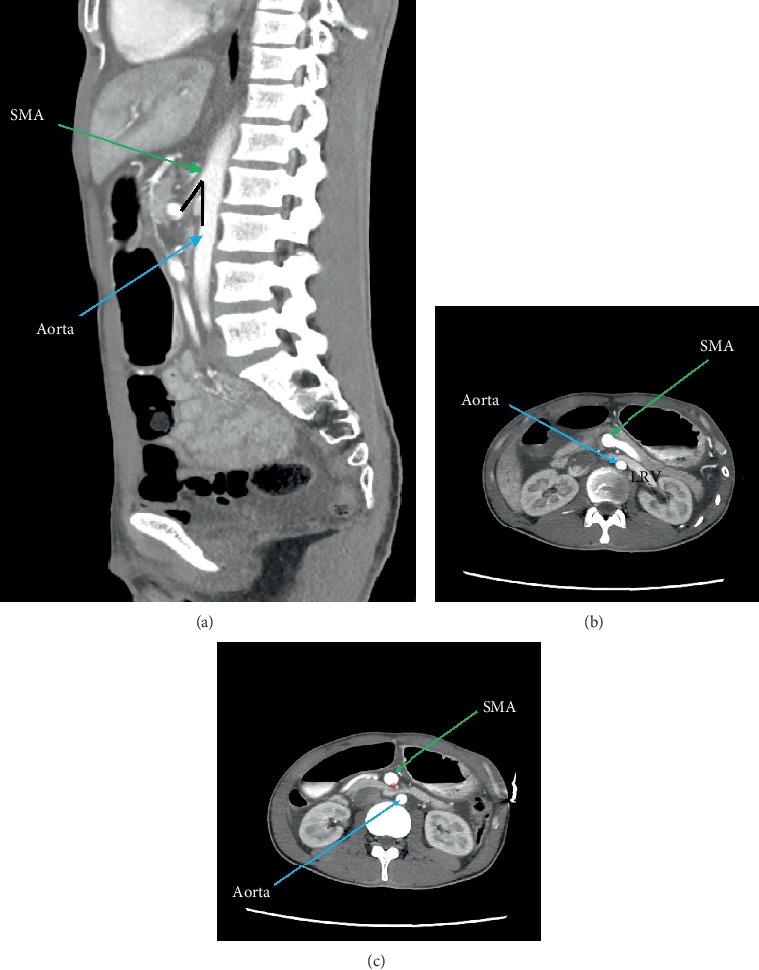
Contrasted CT abdomen image in (a) showing the narrow-angle created by the SMA anteriorly and descending aorta posteriorly. (b) and (c) showing dilated left renal vein (LRV) and third D3 (marked by^*∗*^), respectively, which are compressed by descending aorta posteriorly and SMA anteriorly.
